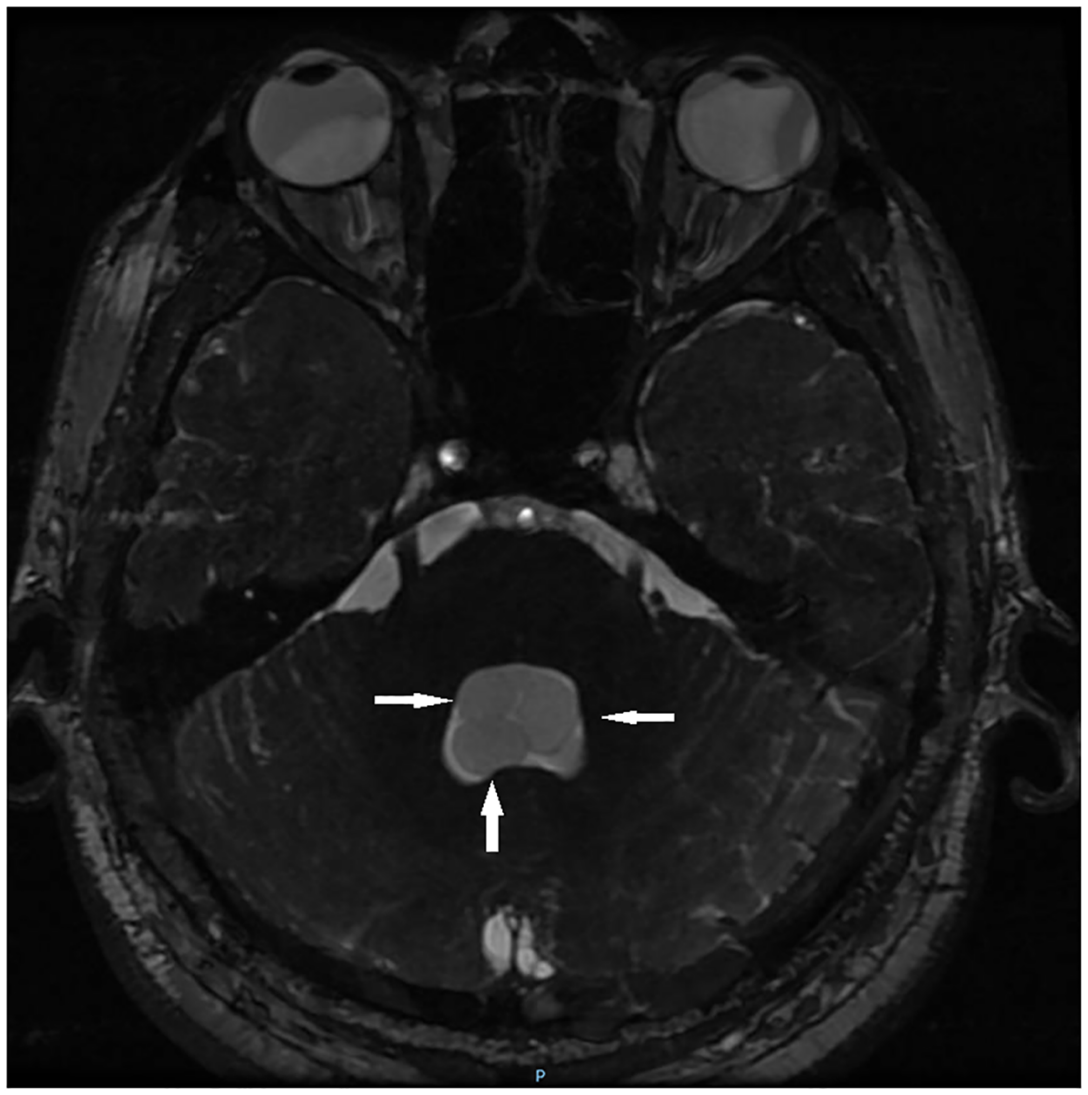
Example of multiple cysticerci located in the fourth ventricle (MRI FIESTA).
